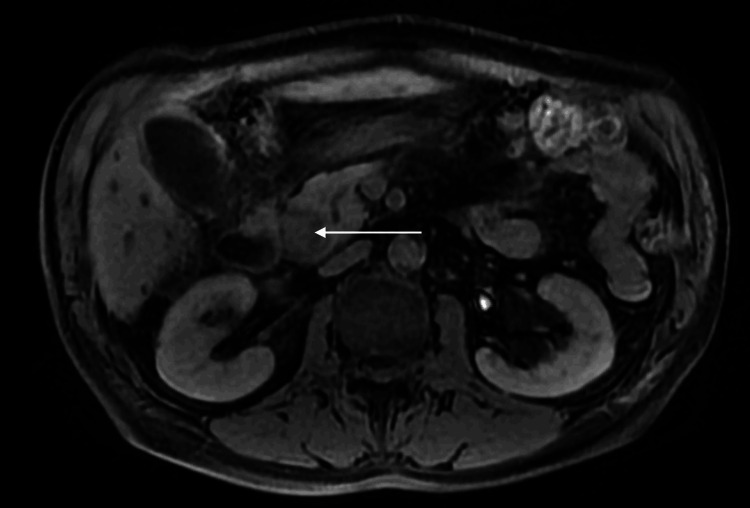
Axial T1-weighted fat-saturated image. Axial T1-weighted fat-saturated image acquired after intravenous gadolinium administration during the late venous phase displays a hyperintense lesion in the distal common bile duct (arrow).
